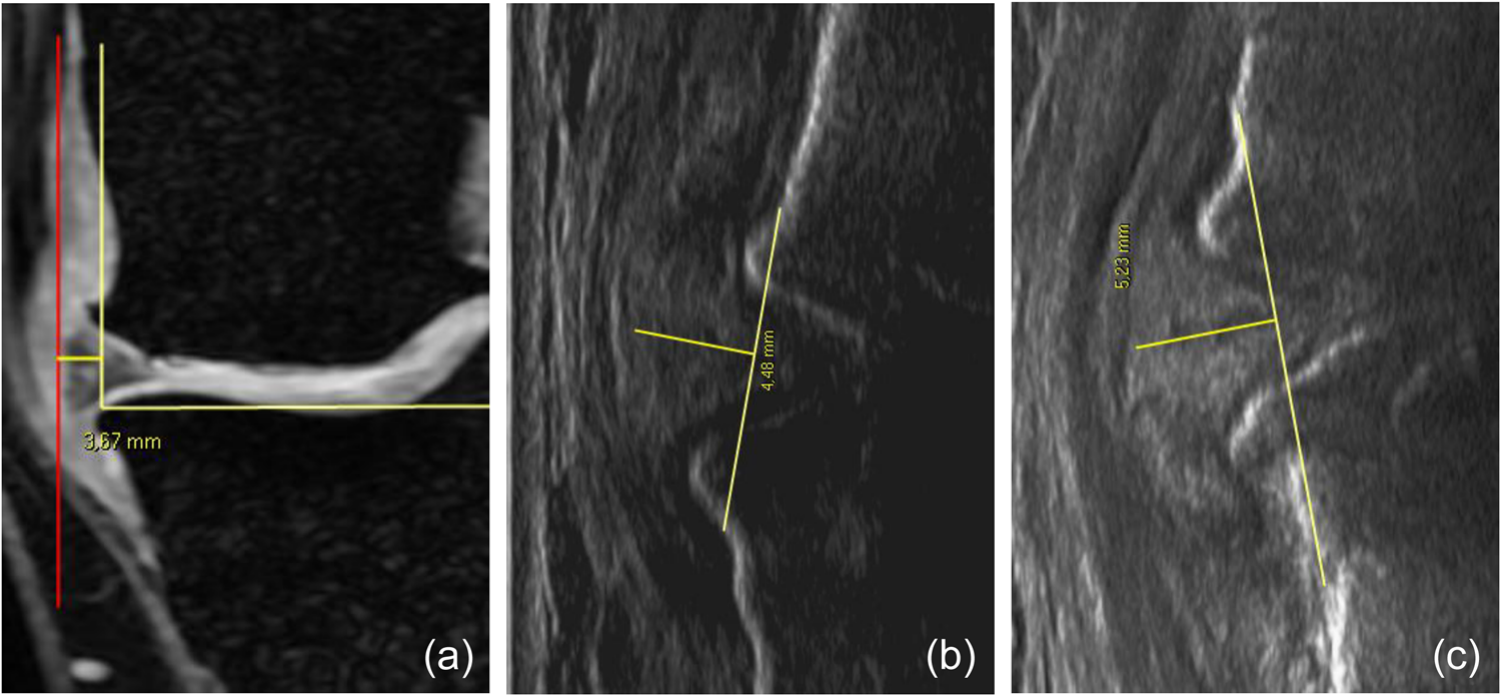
Measurement of meniscal extrusion in a male patient (52 years) using magnetic resonance (a), ultrasound in clinostatism position (b) and ultrasound in orthostatism position (c).
